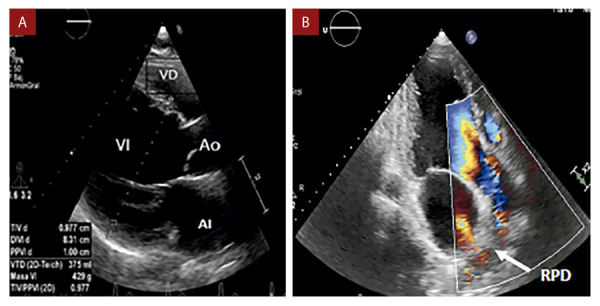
Ecocardiografía transtorácica. A) Vista para esternal eje largo: Dilatación severa de cavidades cardiacas izquierdas (diámetro telediastólico de ventrículo izquierdo: 83 mm). B) Vista apical 3 cámaras: Se aprecia regurgitación aórtica al colocar doppler color y nacimiento de rama pulmonar derecha a partir de pared posterior de aorta ascendente. RPD: rama pulmonar derecha
